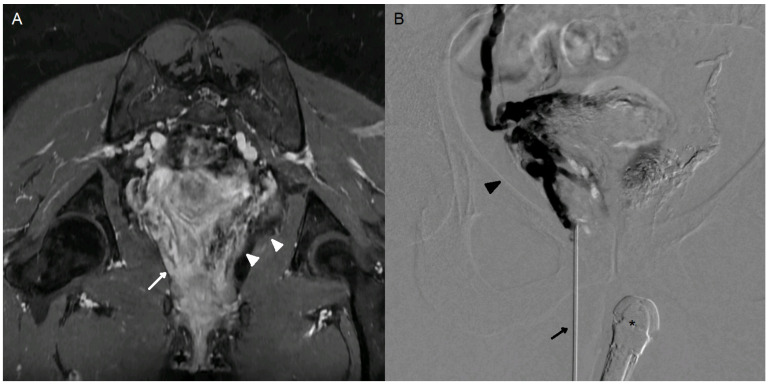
A 35-year-old woman (case n°6), G1P1, presenting with a 3-year history of chronic pelvic pain. Significant improvement in symptoms was achieved following endovascular embolization. However, dyspareunia (VAS 7/10) and postcoital pain (VAS 6/10) persist. (A) Oblique coronal MRI in the vaginal axis, LAVA FS sequence following gadolinium-based contrast injection (GE Systems, Signa Hero), demonstrating persistent right perivaginal varices (white arrow). The embolic agent (Onyx) is clearly visualized on this 3D gradient-echo sequence as a susceptibility artifact in the left perivaginal varices (white arrowhead). (B) Percutaneous puncture of right perivaginal varices (dark arrowhead) with a n18-gauge needle (dark arrow) under endovaginal guidance (*). Slow injection of iodinated contrast agent demonstrating opacification of the entire perivaginal and peri-uterine variceal network on the right side.
